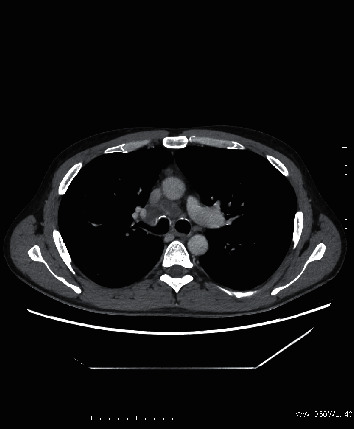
Axial contrast-enhanced CT scan with the presence of enlarged, necrotic mediastinal lymph nodes at the level of carina.
